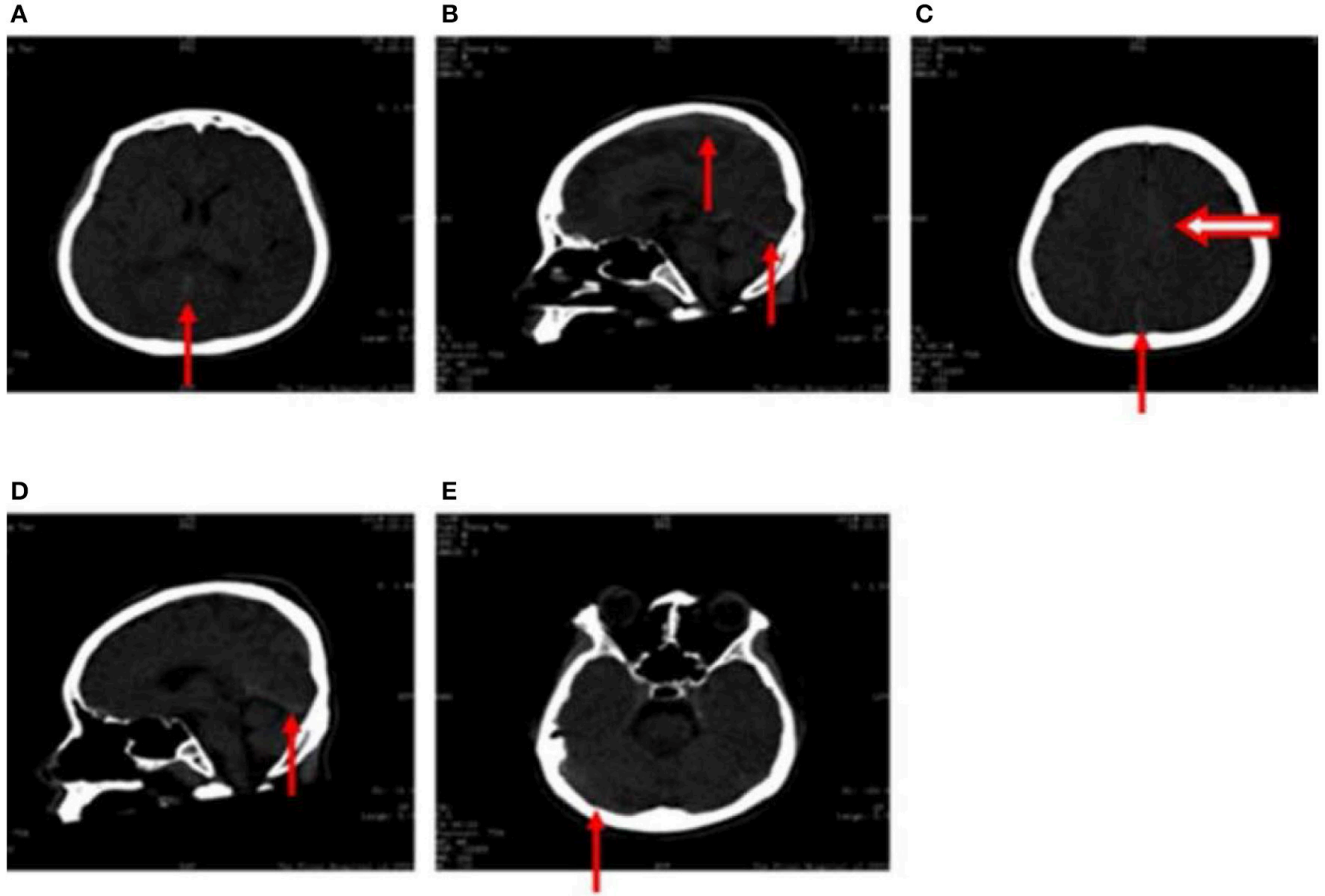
(A–E) Computed tomography images obtained following hospitalization show high-density signals in the great cerebral vein (arrow in A), sagittal sinus (arrow in B,D), torcular herophili (arrow in C), transverse sinus (arrow in E) (thrombus formation was not excluded). High-density signals were also observed in the cerebral falx (hollow arrow in C), leading us to consider subarachnoid hemorrhage.
